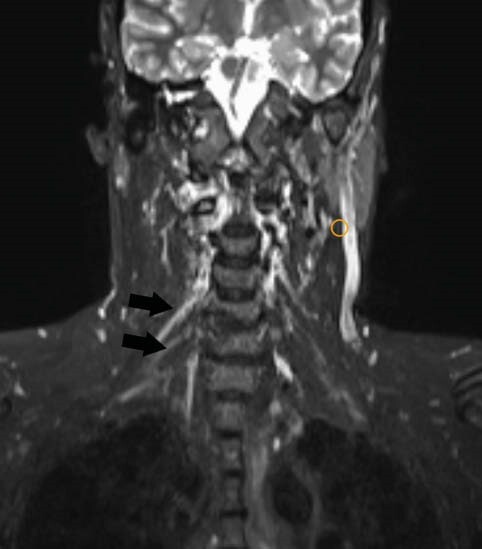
Figure 4. Axial CT chest section in lung window showing pneumothorax (white arrow) and pneumatocele ( grey arrow) with peripheral ground glass opacities and consolidations in both lungs.
